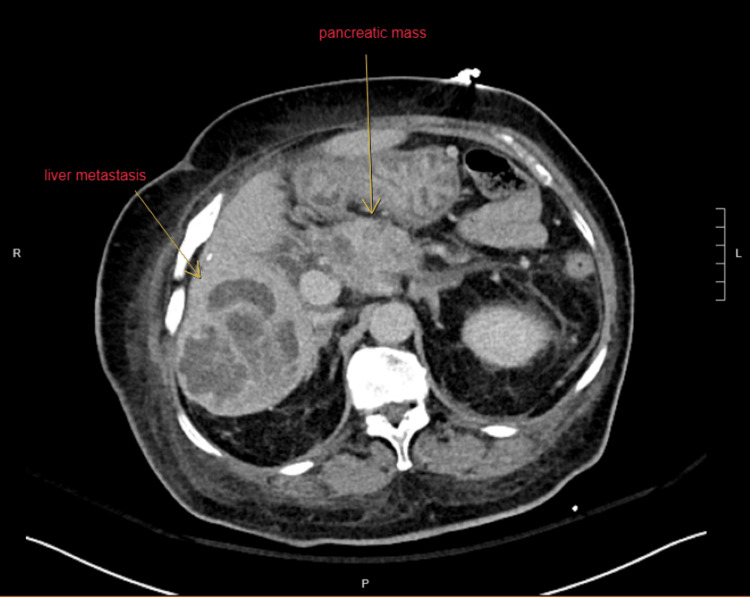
Computed tomography scan of the abdomen showing the pancreatic mass with multiple liver metastases, confirming the presence of advanced pancreatic cancer
